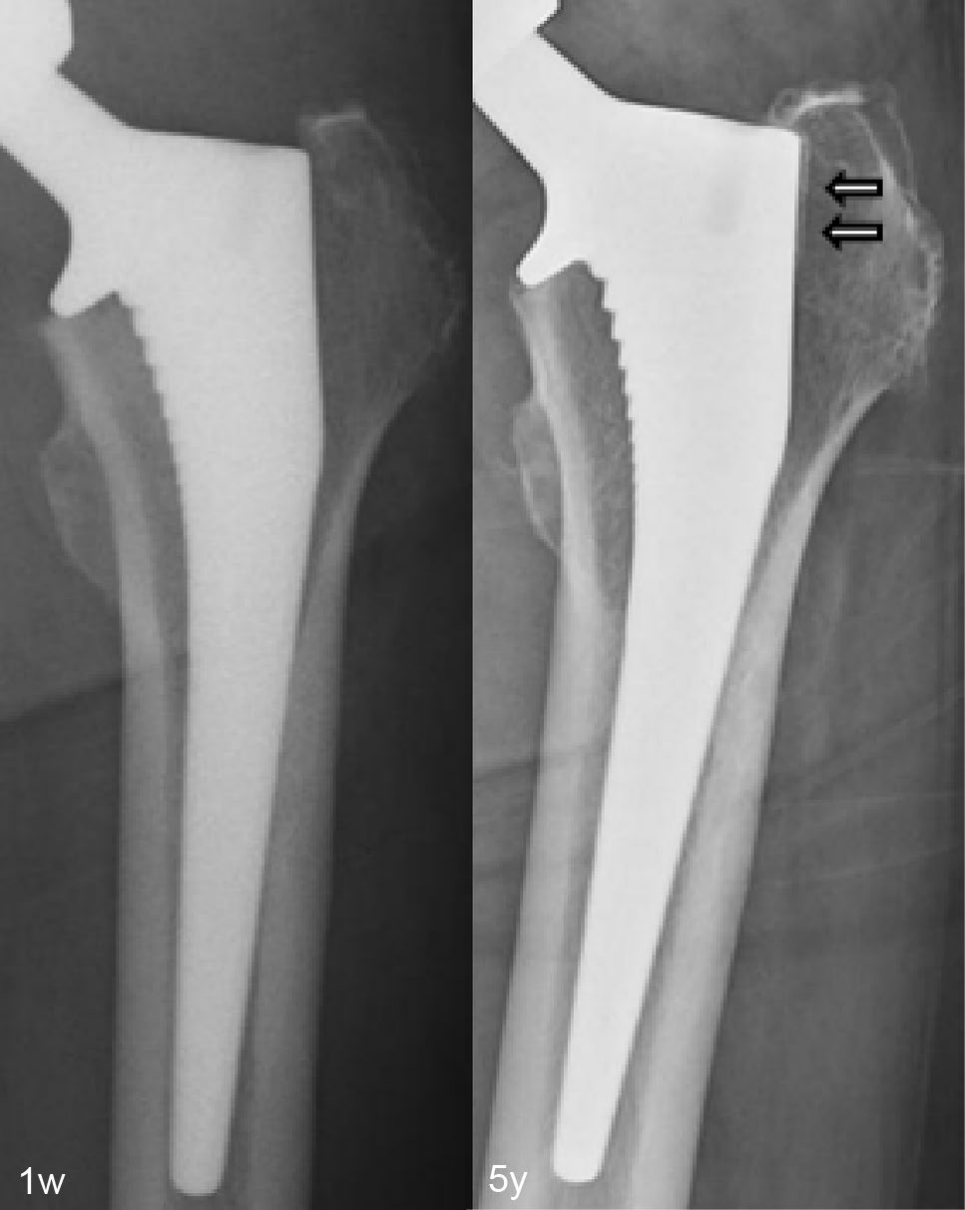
Longitudinal hip radiographs of an 81-year-old female patient who had THA using CORAIL^®^ collared stem with bone graft showing evidence of reactive lines (arrows), but no stress shielding, 5 years after surgery
